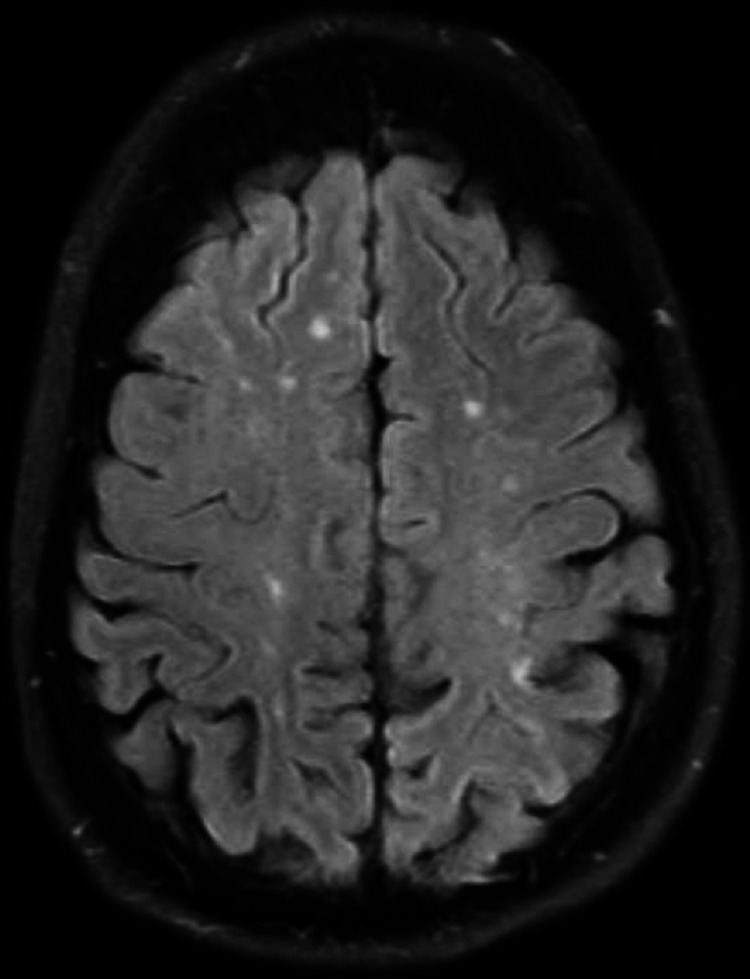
T2 sequence of brain MRI with contrast showing bilateral cerebral hemispheres
